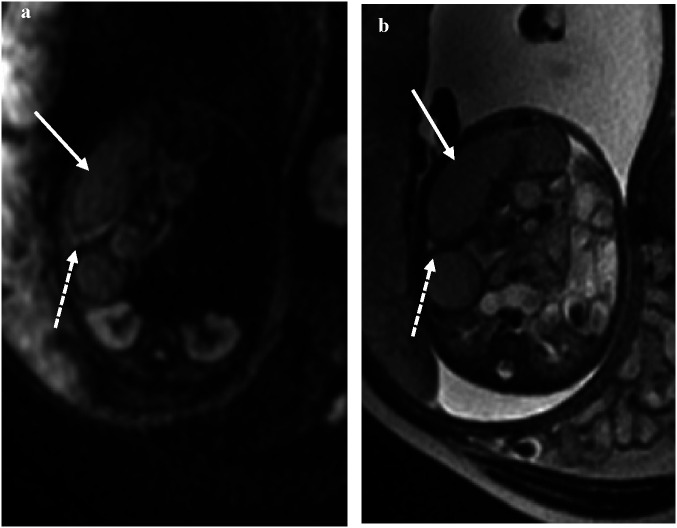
At 35 weeks of gestation, (a) the DWI signal intensity of the obstruction of the intestine is higher than that of the lumen, suggesting ischemia. (b) The corresponding intestinal lumen showed slightly lower signal intensity on T2WI.
